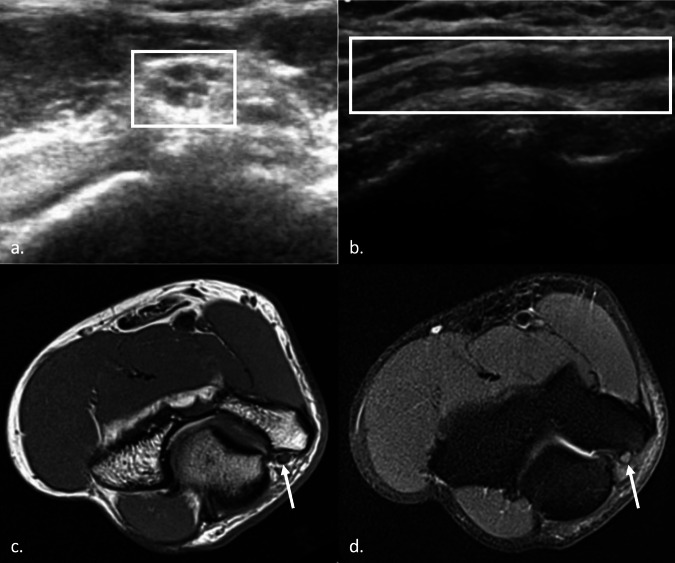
Normal nerve appearance on US and MRI examination. Transverse axis US image (a) and longitudinal axis US image (b) of UN at the level of the elbow joint. The nerve has a honeycomb appearance in the short axis (a) and a coarse hypoechoic appearance in the long axis (b). T1 (c) and fat-suppressed PD (d) MRI images of UN (arrow) in the cubital canal. The nerve appears isointense to muscle in c and mildly hyperintense in d
